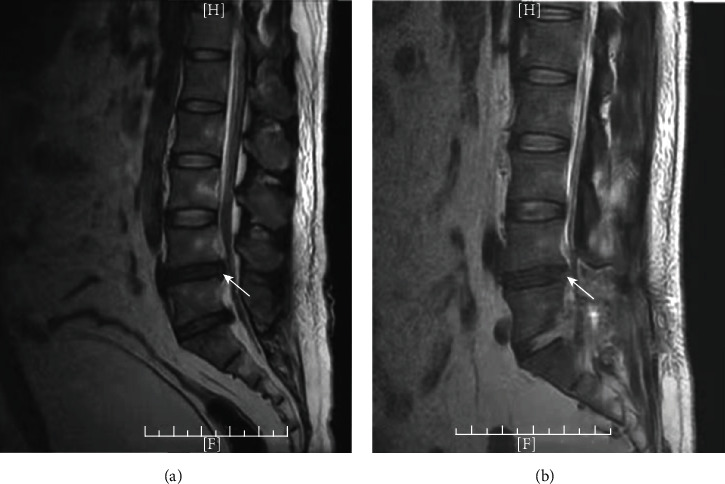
Comparison of magnetic resonance images before and after physical therapy. Compared with the image before treatment (a), disc herniation (arrow) after treatment (b) decreased significantly.
